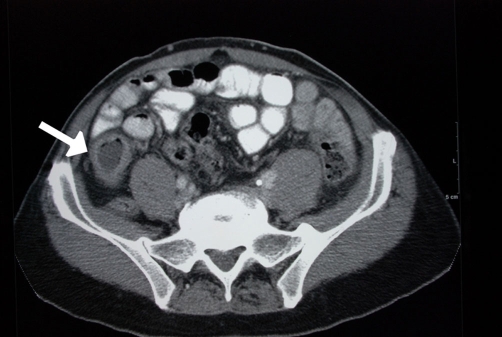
Abdominal CT demonstrating a stricture of intestinal tuberculosis at ileal region (arrow).
